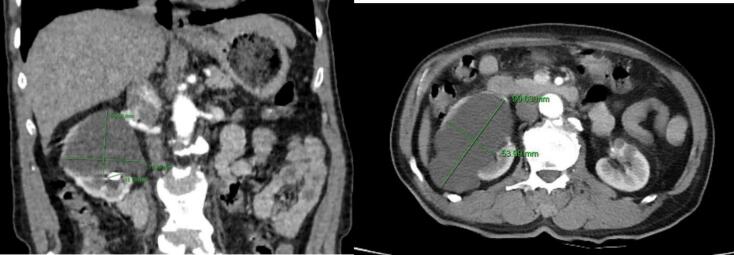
Image of the right renal cyst and renal calculi on CT scan of patient 2.
